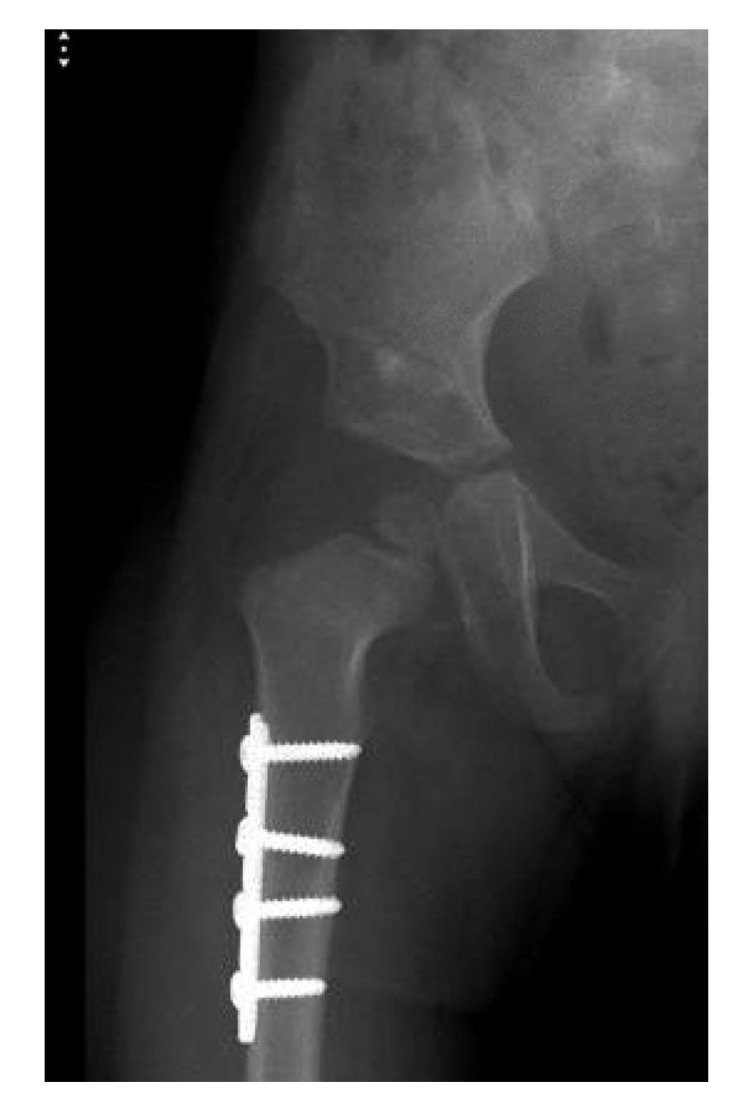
Four months postoperative radiograph showing intact Shenton line.
